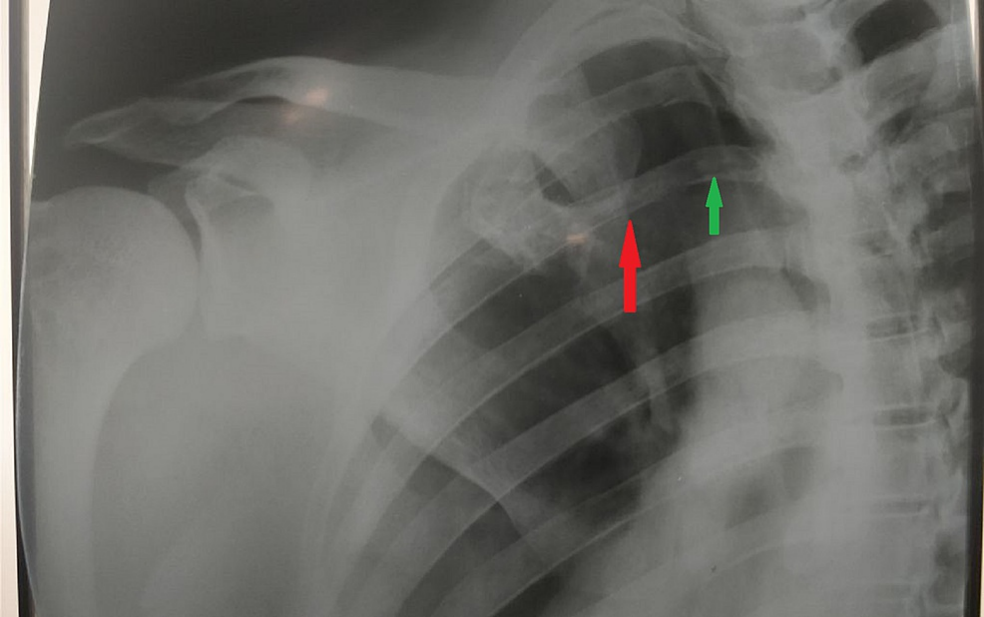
Digital radiograph showing the dislocation of the sternoclavicular joint with separation between the medial end of clavicle (red arrow) and sternum clearly visible (green arrow).
